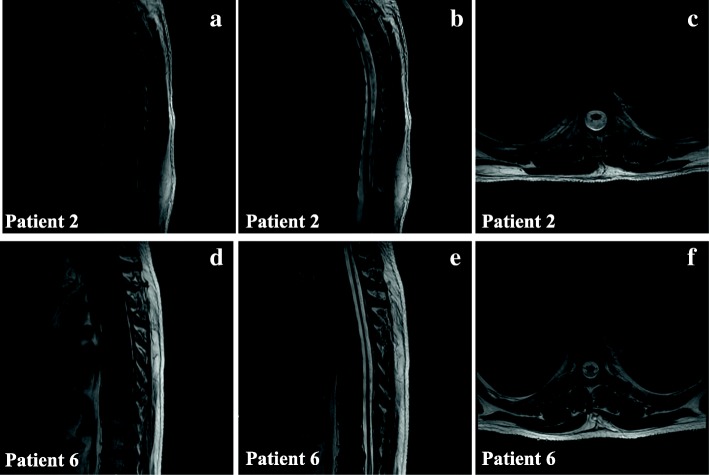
Spinal MRI scans of patient 2 and patient 6 show diffuse cord atrophy. a Sagittal T1-weighted images of patient 2. b Sagittal T2-weighted images of patient 2. c Axial T2-weighted images of patient 2. d Sagittal T1-weighted images of patient 6. e Sagittal T2-weighted images of patient 6. f Axial T2-weighted images of patient 6
